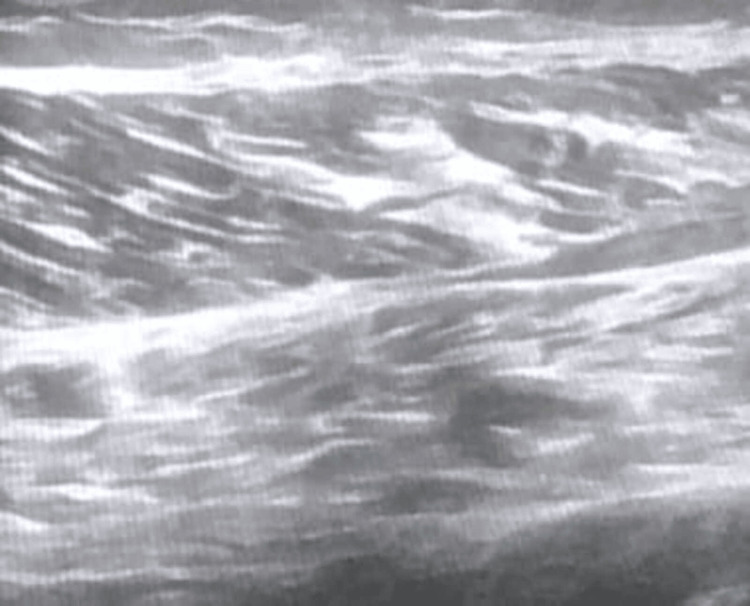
Postoperative ultrasonography image of the calf region The previously seen cyst with scolex was not observed in the postoperative ultrasonography picture.
